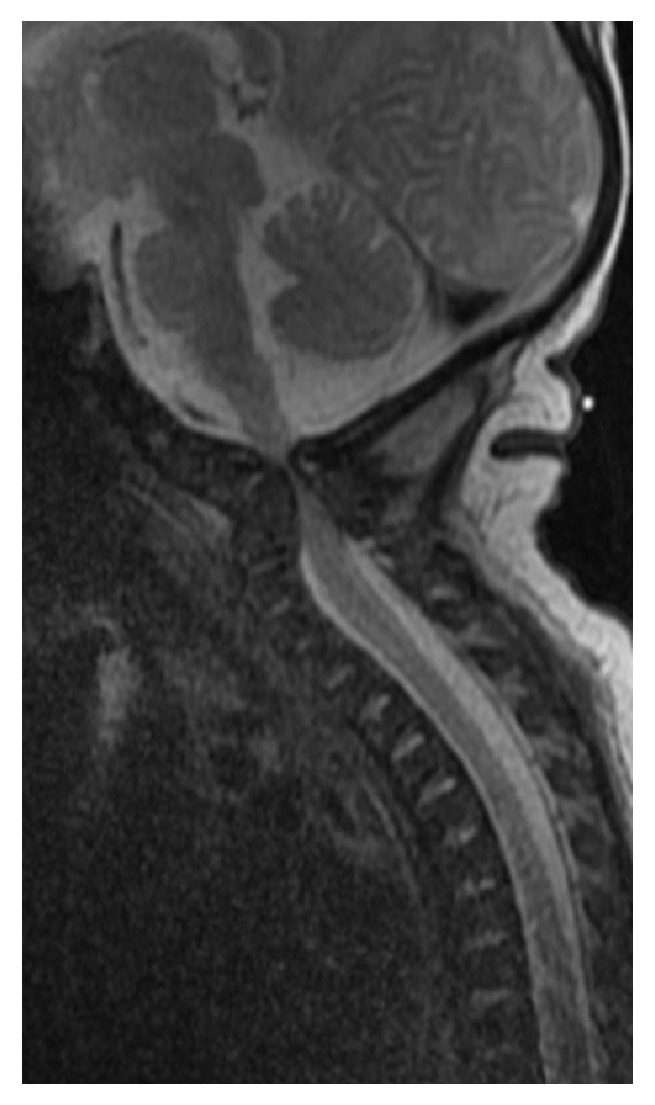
MRI of cervical spine: spinal cord compression at level of the foramen magnum secondary to craniocervical junction anomaly with focal severe kyphosis of the upper cervical spine (C3-C4).
